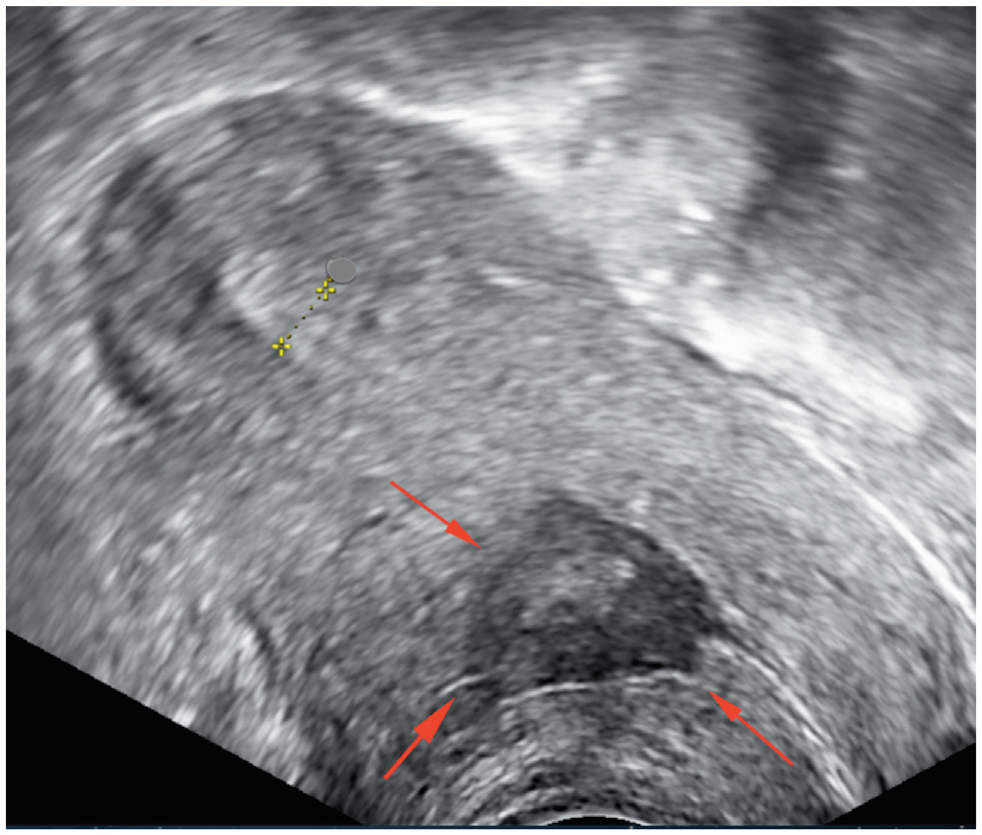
Pelvic ultrasonography before treatment shows a heterogenic cavity at the site of cesarean section.
